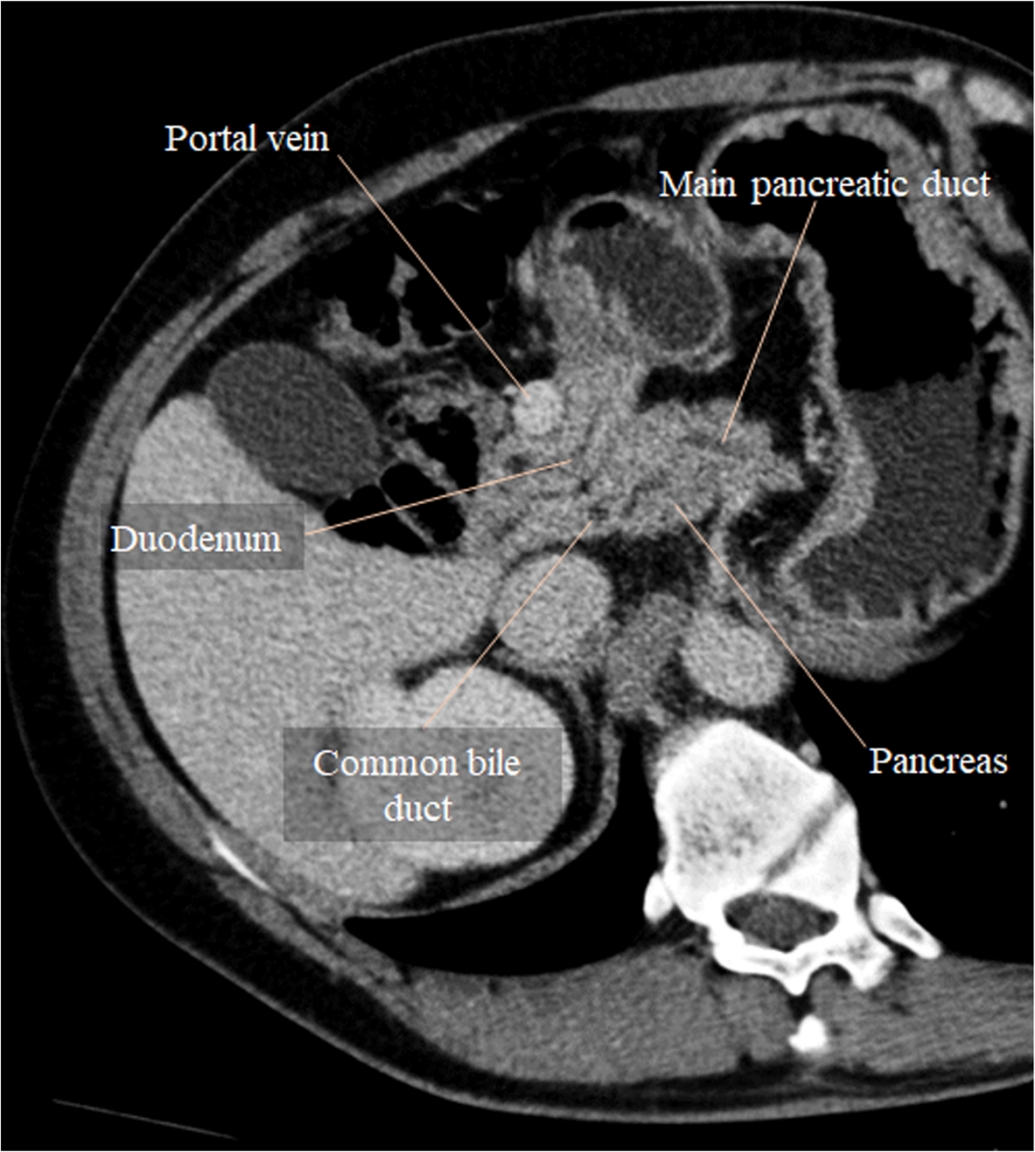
Preduodenal PV. MPR image from portal venous phase CT shows the main PV coursing in front of the duodenum and pancreas, an incidental finding in this case
